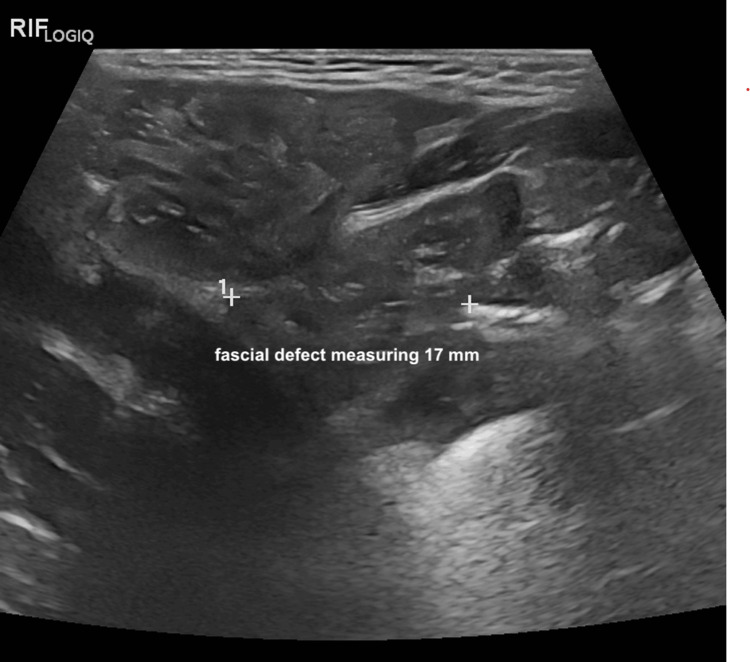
Ultrasound of the abdominal wall showing a fascial defect consistent with hernia Ultrasound of the right iliac fossa demonstrates a 17 mm fascial defect suggestive of an abdominal wall hernia, with possible herniation of fat or bowel through the defect. RIF: right iliac fossa
